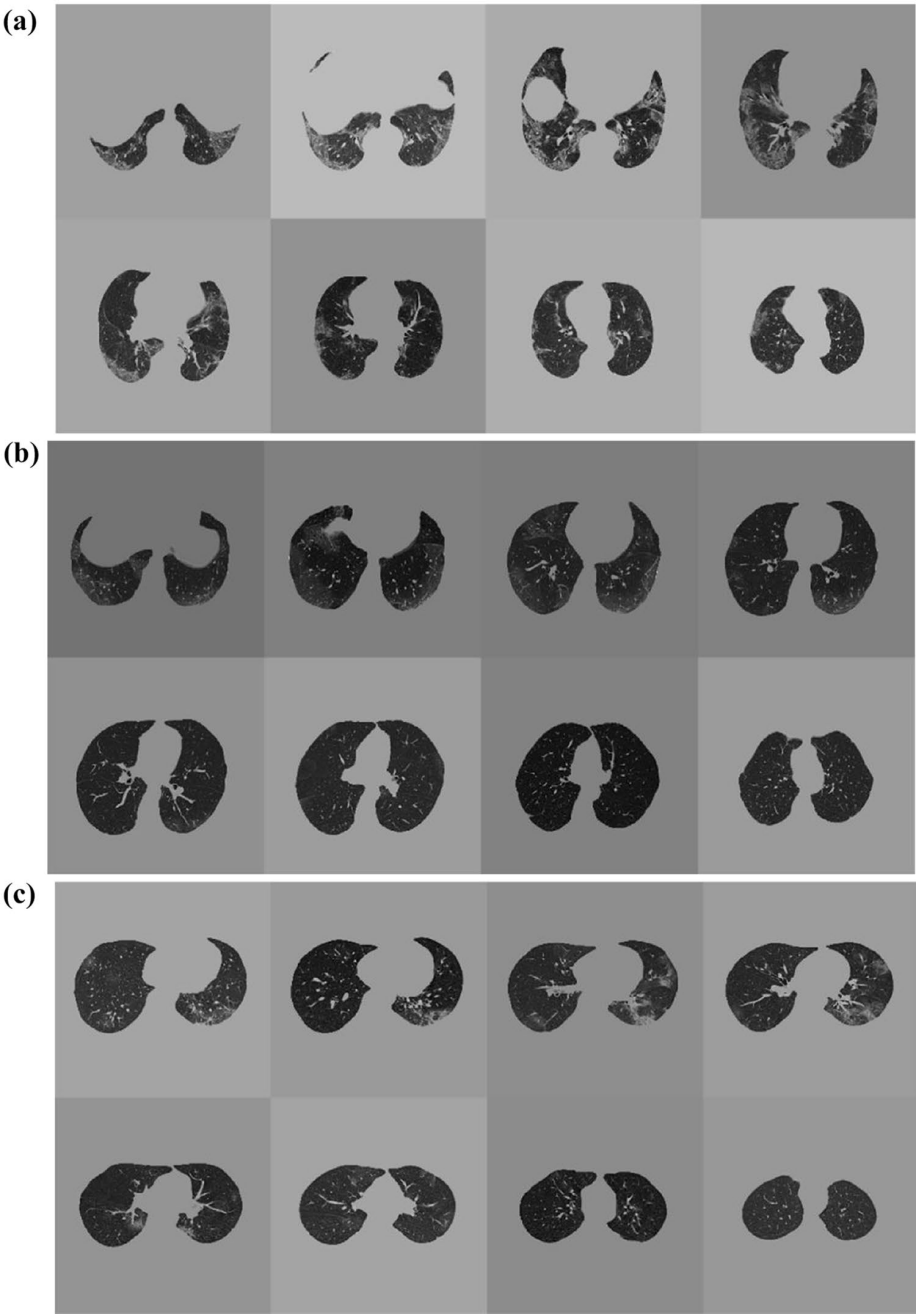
CT examples of patients correctly predicted in a survivor, b intubation, and c ICU admission prediction
